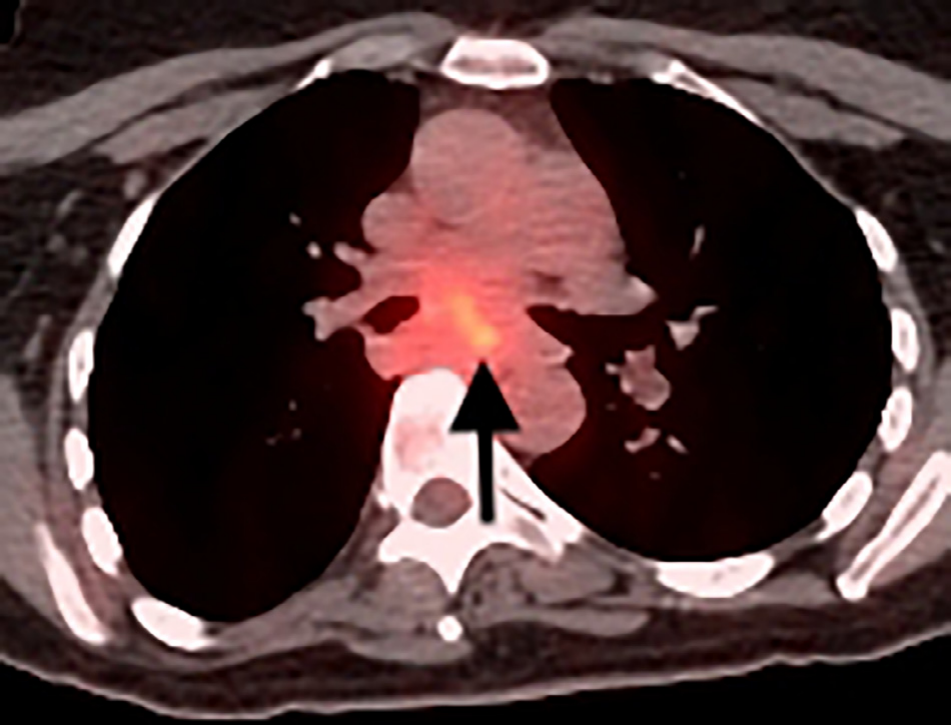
PET/CT demonstrating FDG-avid subcarinal lymphadenopathy (arrow).
